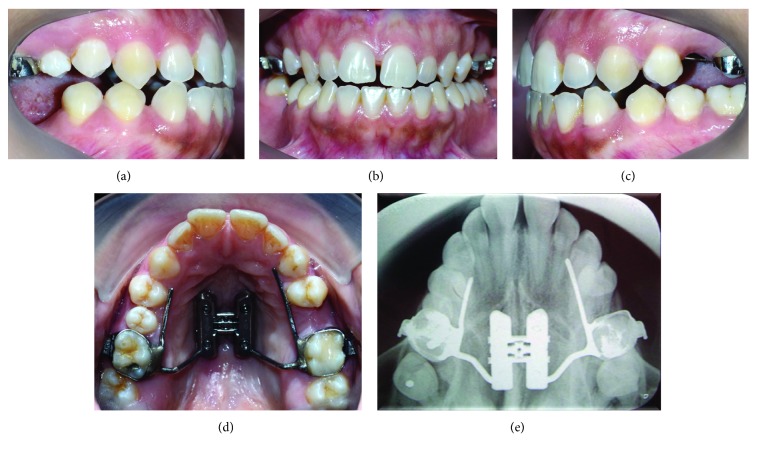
Images of the result after the corticopuncture procedure and second activation protocol: (a) right, (b) frontal, (c) left, (d) upper occlusal view after opening of the medial palatine suture, and (e) upper occlusal X-ray.
